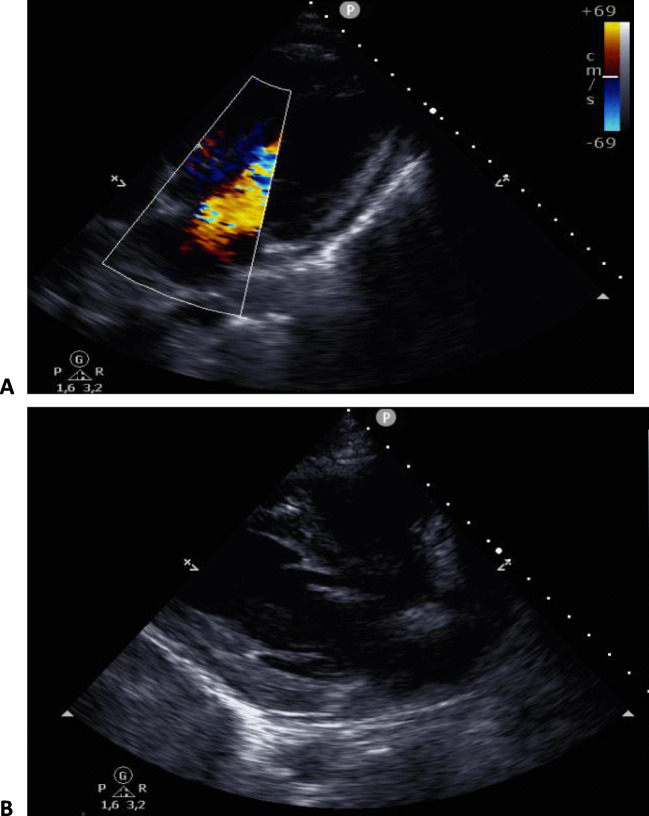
Images of echocardiogram at hospitalization day 2; suspicion on cardiac involvement as part of MIS. The panels below demonstrate depressed systolic left ventricular function, slight tricuspidal valve insufficiency, aotic valve with 3 leaflets without pericardial effusion, and a dilated vena cava inferior
